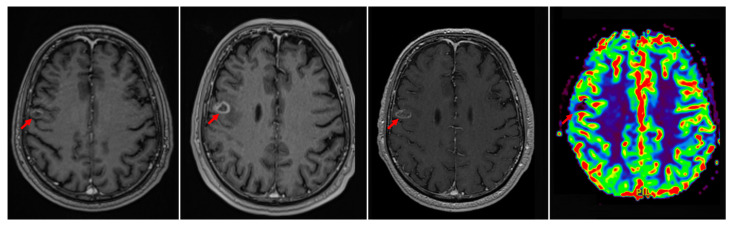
Longitudinal axial post-contrast T1-weighted MRI follow-up of a patient with a lung cancer brain metastasis treated with single-fraction stereotactic radiosurgery, with examinations acquired on different MRI scanners at 14, 18, and 24 months after treatment. Serial imaging demonstrates progressive dimensional enlargement of the treated lesion. The relative cerebral blood volume (rCBV) map obtained at the final follow-up shows no focal areas of increased perfusion, supporting radiation-induced changes rather than tumor recurrence despite interval lesion enlargement.
